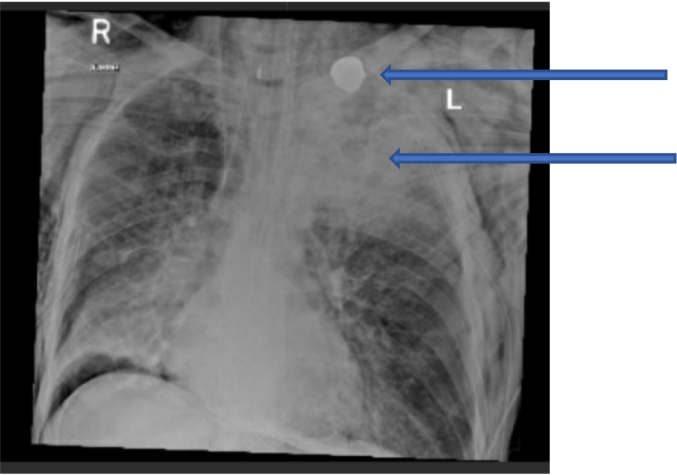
Chest X-ray revealing a foreign body in the left upper chest with significant contusions for case 2 (upper arrow: foreign body; lower arrow: lung contusion).
